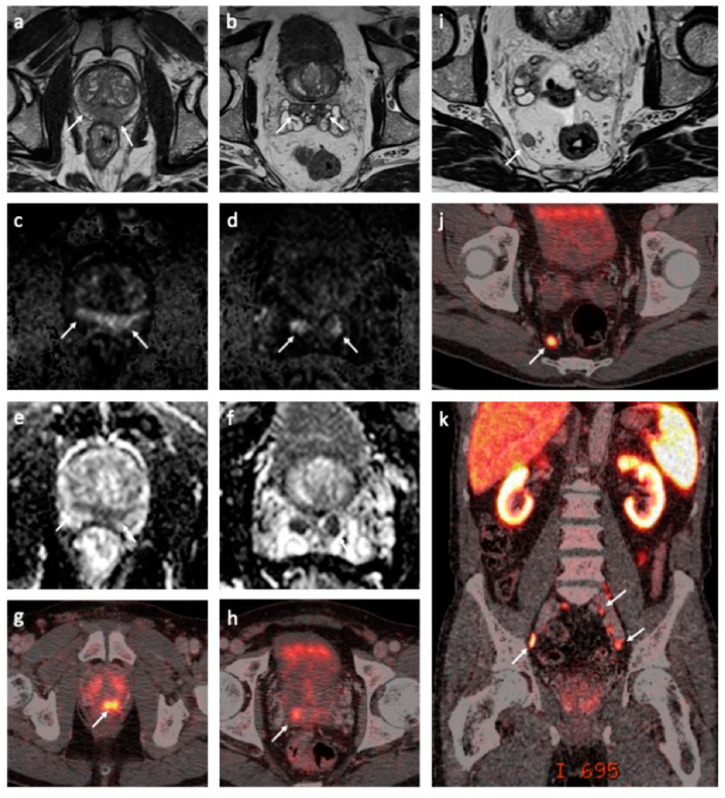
Magnetic resonance imaging (MRI) and prostate-specific membrane antigen (PSMA)-positron emission tomography (PET)/computed tomography (CT) findings at the baseline. Axial T2-weighted MRI sequences demonstrated a large, diffuse infiltrating tumor in the peripheral zone with seminal vesicle invasion ((a,b), white arrows). The tumor was hyperintense on diffusion-weighted MRI (DW-MRI) (b = 1500 s/mm^2^) ((c,d), white arrows) with a corresponding low apparent diffusion coefficient (ADC) (ADC = 0.6 × 10^−3^ mm^2^/s) ((e,f), white arrows). Corresponding tracer uptake on fused PSMA-PET/CT images ((g,h), white arrow). A large lymph node metastasis in the mesorectal fat is shown on the axial T2-weighted MRI ((i), white arrow) and fused PSMA-PET/CT images ((j), white arrow). A coronal-fused PSMA-PET/CT image demonstrates multiple metastases to the pelvic lymph nodes ((k), white arrows).
